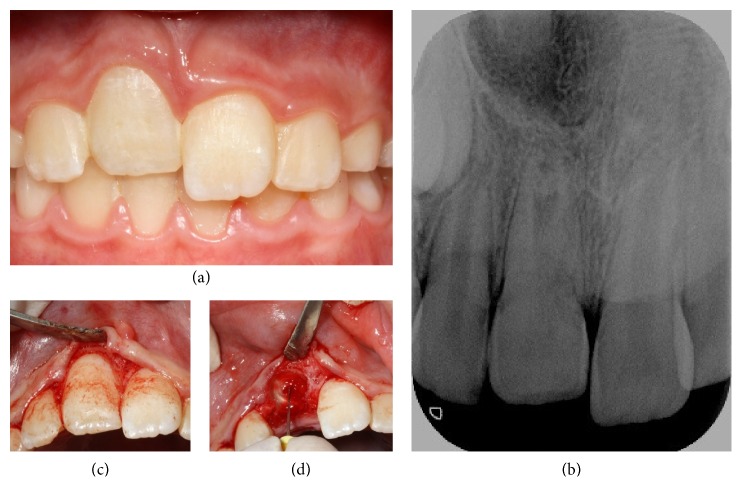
(a) Frontal intraoral photograph of the patient after a trauma to the upper left central incisor [11], which occurred 2 weeks earlier and caused an avulsion that was immediately reimplanted. Observe infraocclusion with respect to 2.1 and 1.2. (b) Periapical control radiograph performed 2 weeks after reinsertion of 11, in which intrusion and ankylosis are apparent. (c) Intraoral photograph during decoronation as the full-thickness flap is raised around the ankylosed tooth. (d) Intraoral photograph during decoronation, as the pulp tissue is removed from the root canal using an endodontic K-file.
